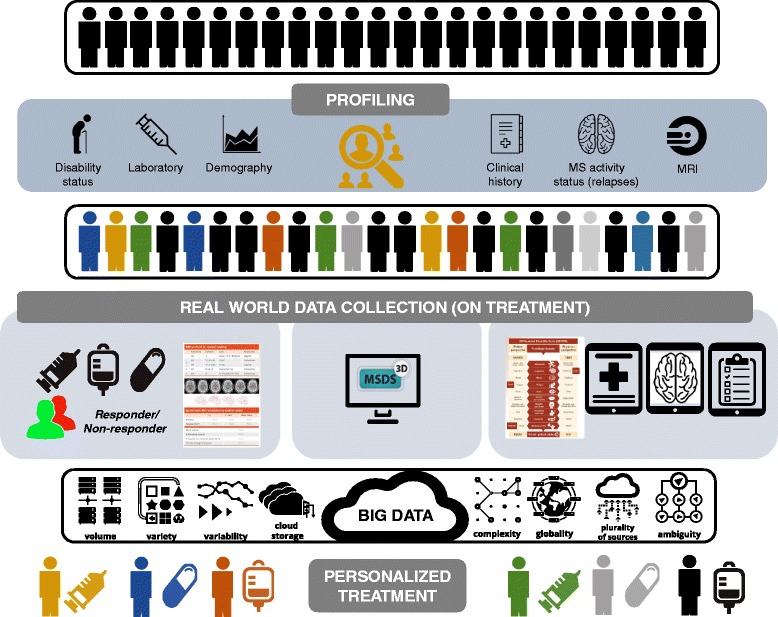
Profiling patients and collecting data. As part of the personalized medicine approach, MS patients should be characterized by a detailed clinical profiling which is the base of all long-term documentation. All patients should be documented during the course of the disease to understand treatment response, document relapses and disease progression as well as quantitative inflammatory and neurodegenerative magnetic resonance imaging (MRI) markers. Physician- and patient-centered parameters should be collected by intelligent software systems as MSDS software. MS big data can be used to analyze treatment response patterns and allow personalized treatments. Therefore, the available information (big data MS) of all included MS patients can assist in complex treatment decisions in future
